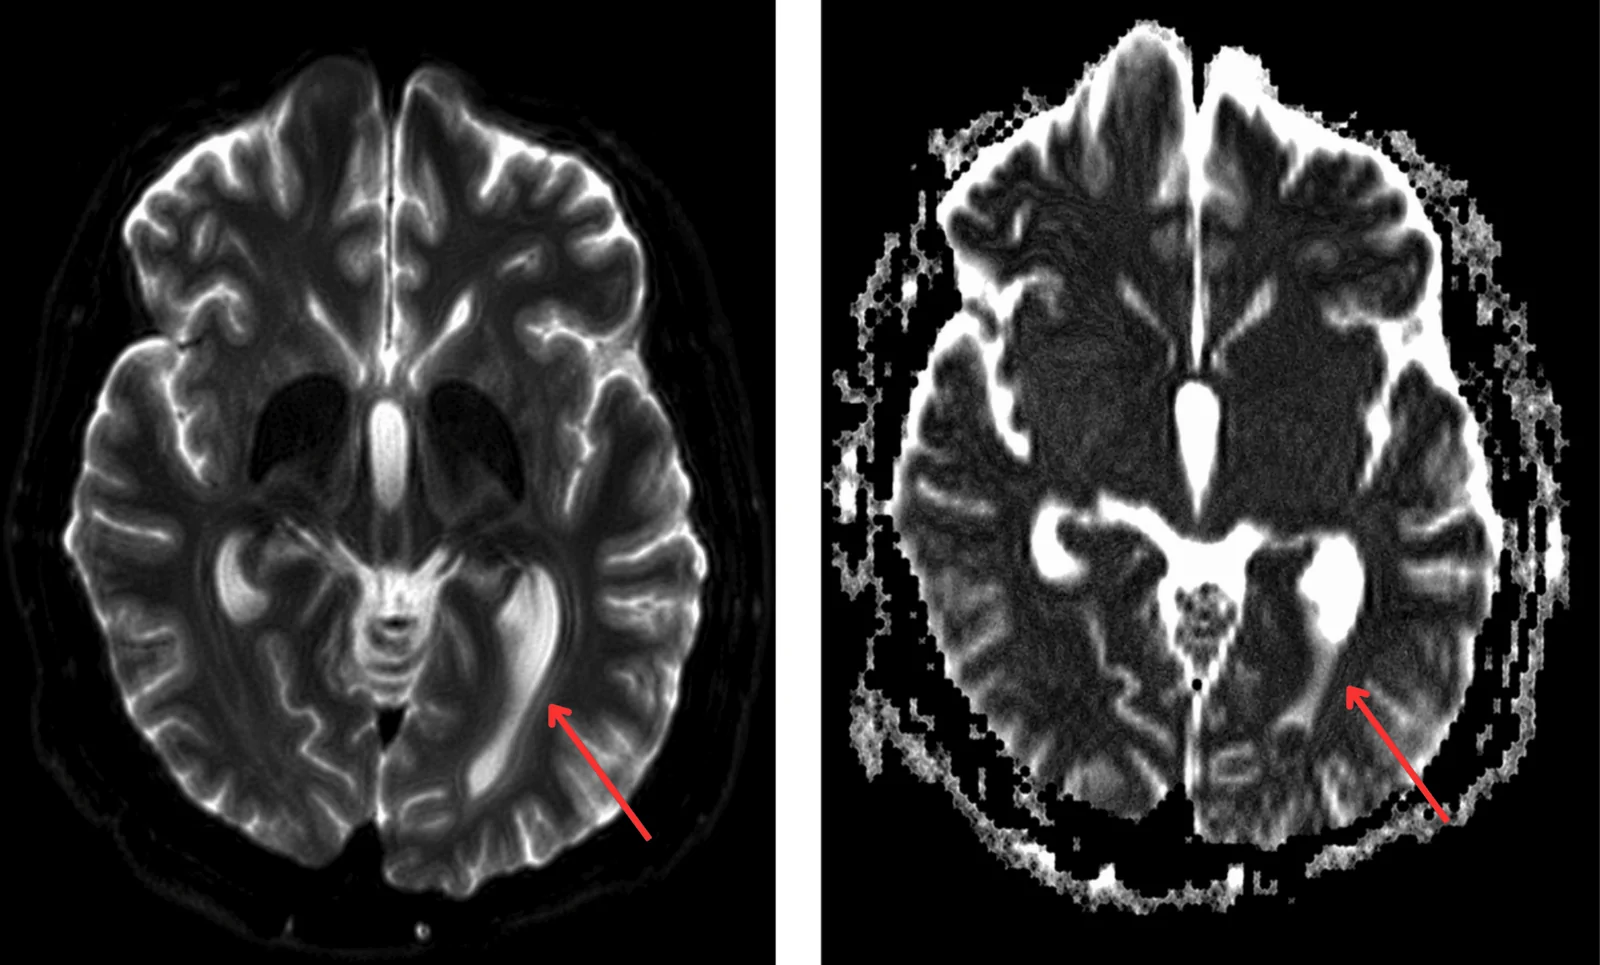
Repeat brain MRI demonstrating persistent tiny foci of restricted diffusion along the occipital horns of the lateral ventricles, slightly more prominent on the left. Diffusion-weighted imaging (DWI) is shown on the left, with the corresponding apparent diffusion coefficient (ADC) map on the right. Red arrows indicate the area of abnormal diffusion.
